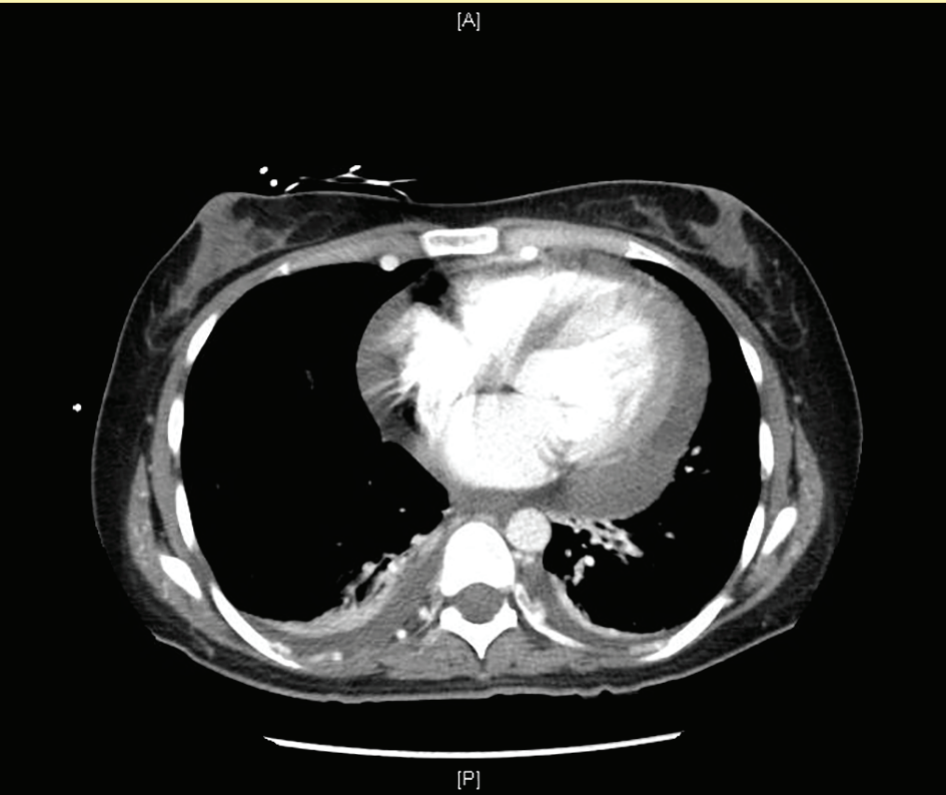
Computed tomography (CT) of chest showing pericardial effusion with pericardial thickening.
